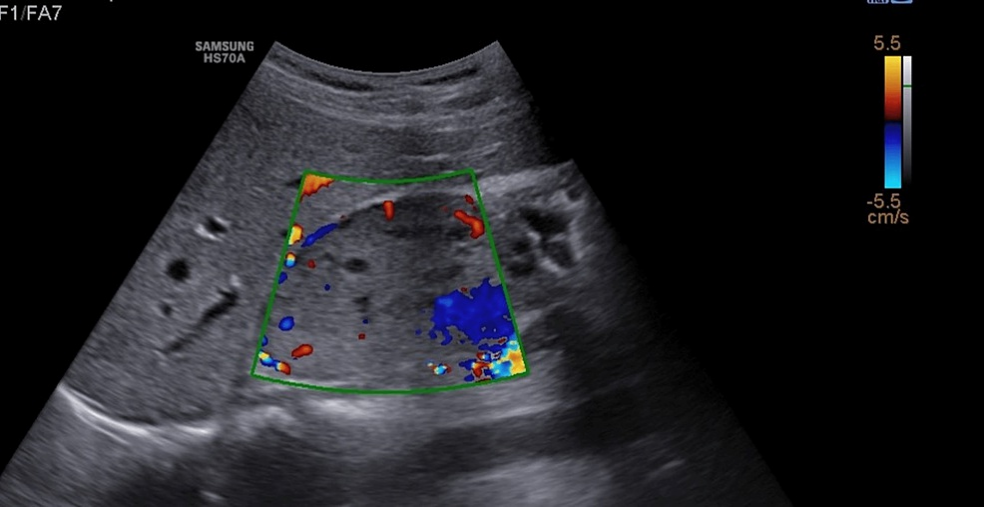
On colour Doppler, moderate peripheral and central vascularity is demonstrated.
